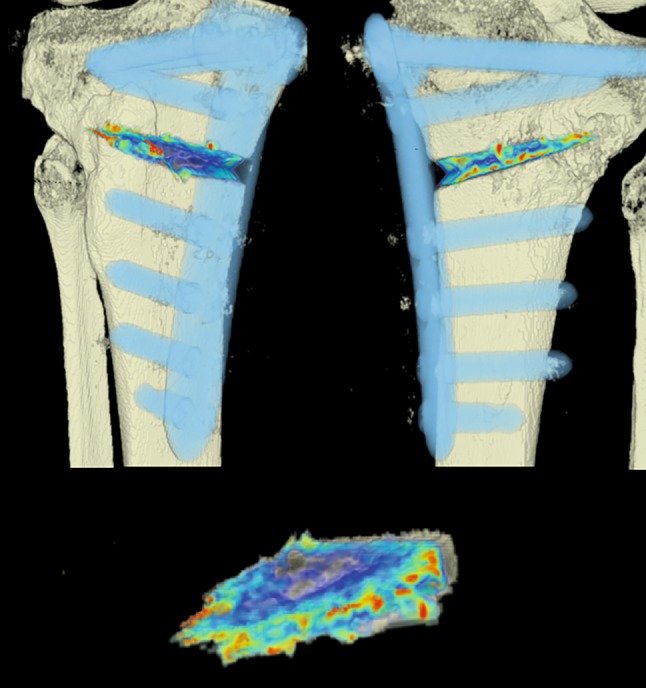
CT scan analysis included a visualization of the highest BMD changes: the gap is evenly filled with new bone; *red zones* mark the areas with most bone growth, *green zones* moderate bone growth and *blue areas* show only minimal changes in BMD
